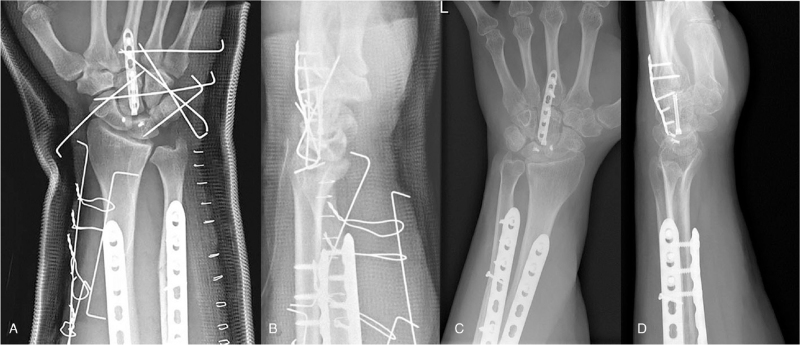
The capitate, CMCJ, and hamate were reduced and fixed. The SL and LT ligaments were repaired using two anchor sutures (black revere triangles), and other intercarpal ligaments were repaired. Transcarpal pins were inserted and a 2.0 small plate was fixed to buttress the CMCJ. Immediate (A, B) and 12 months (C, D) postoperative radiographs demonstrated well reduced carpal alignment. CMCJ = carpometacarpal joint.
